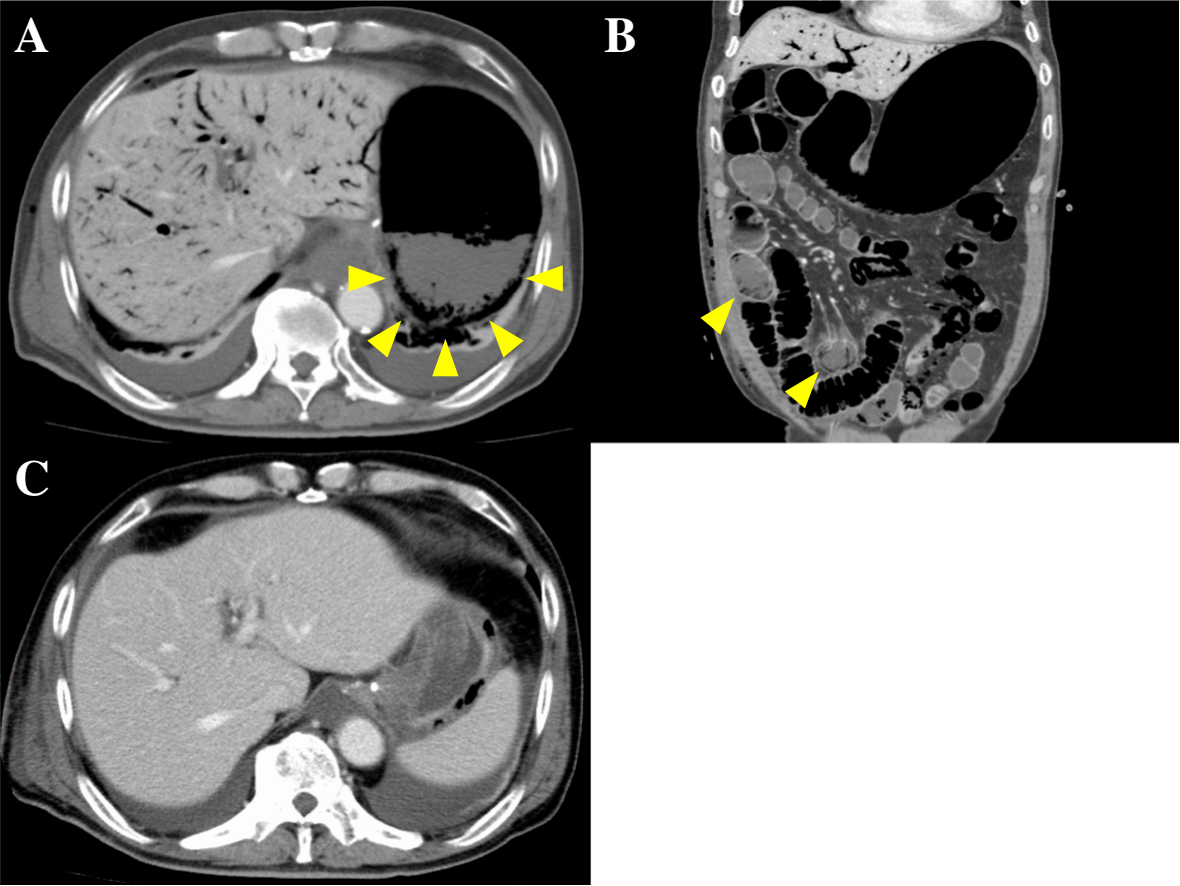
a, b Computed tomography of the abdomen showing massive hepatic portal venous gas and a dilated gastrointestinal tract with pneumatosis intestinalis (*arrowheads*). c Follow-up computed tomography showing a significant reduction of hepatic portal venous gas
